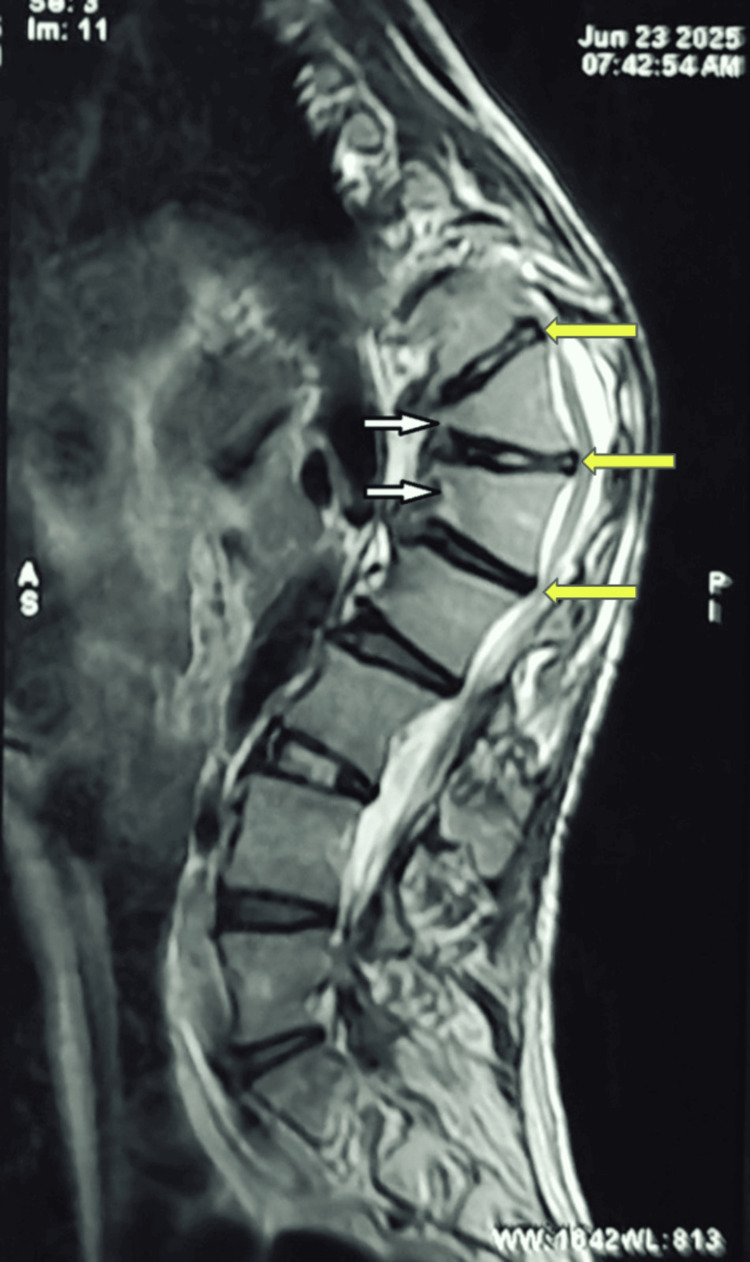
MRI spine for case two showing kypho-scoliotic deformity of dorso-lumbar spine D12 and L1 vertebral body shows anterior wedging (white arrows). D11-D12, D12-L1 and L1-L2 intervertebral discs show mild posterior disc contour bulge (yellow arrows) causing ventral thecal sac indentation
